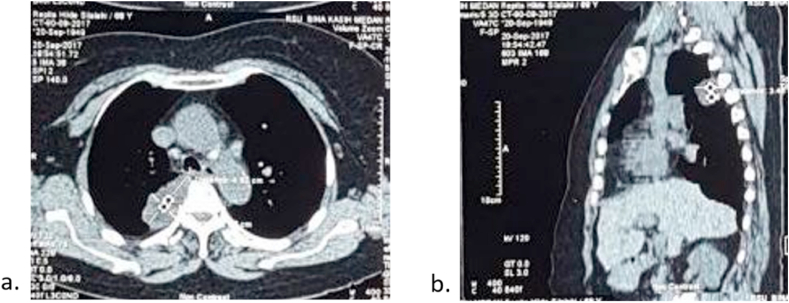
Contrast-enhanced Computed Tomography. (a) axial view (b) sagittal view. Figures (a) and (b) showed a lobulated lesion in the right medial posterior intrathoracic with an attachment to the right 3rd-5th vertebral body and mild right pleural effusion.
